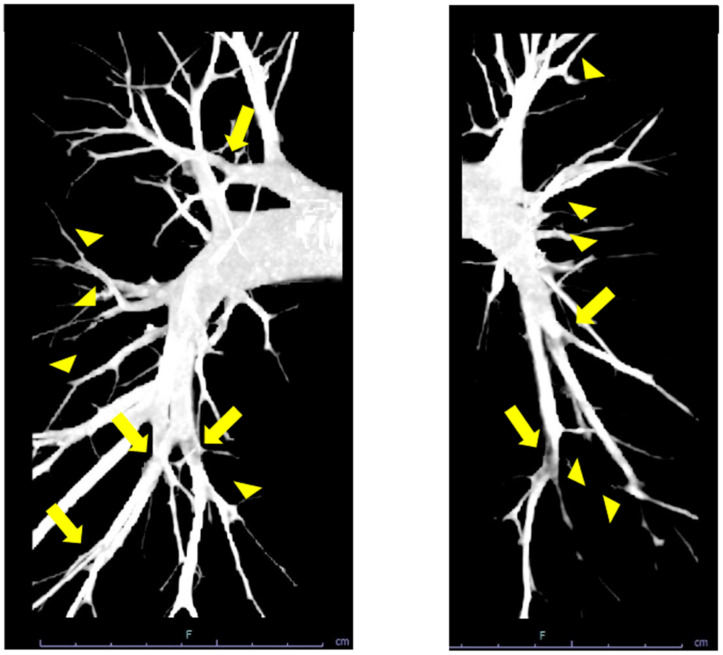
Contrast computed tomography showing web-like strictures (arrows) and abrupt narrowing (arrow heads) in bilateral pulmonary arteries.
